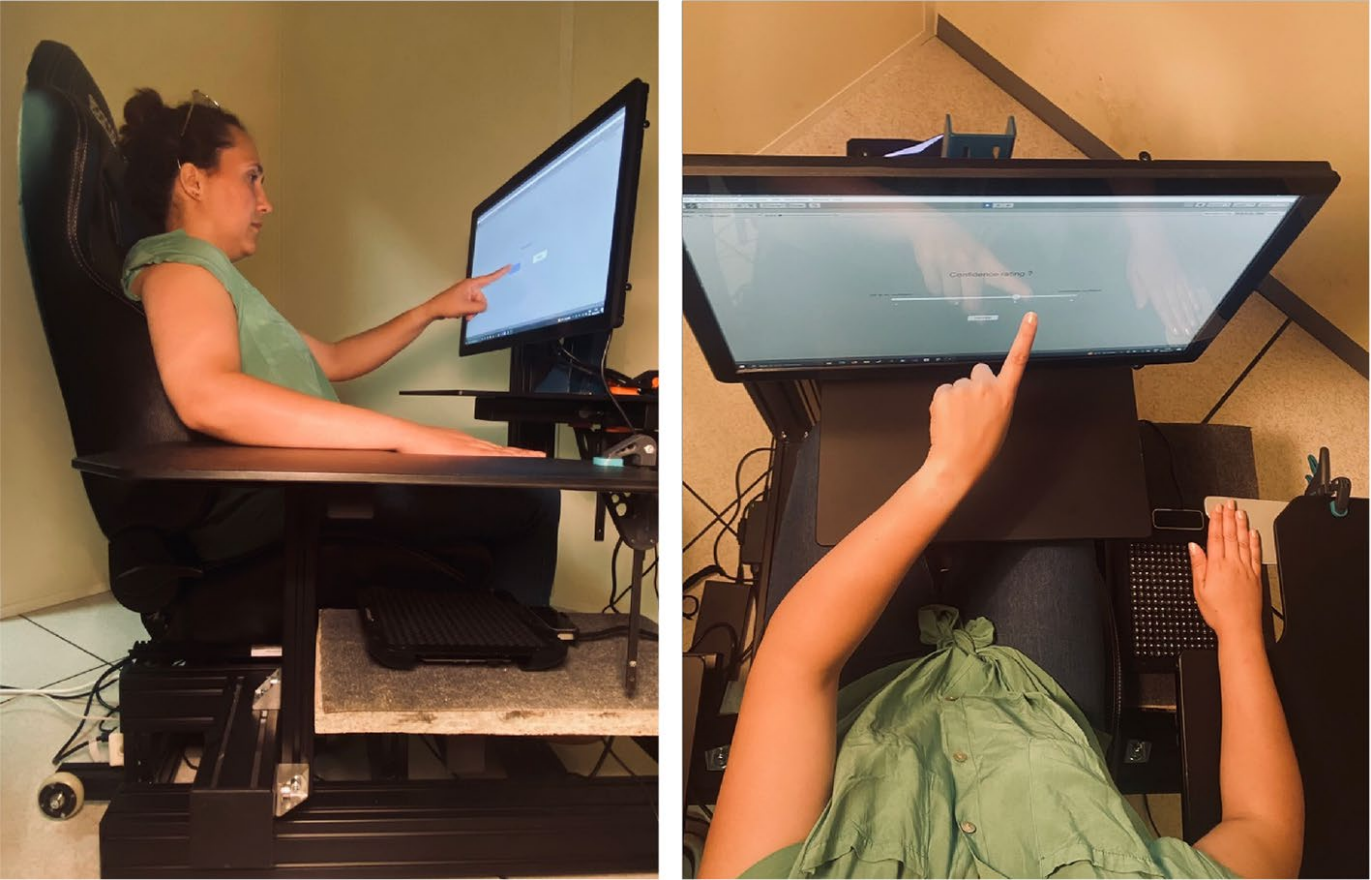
Overview of the experimental setup and participant positioning. Stimuli were delivered using an array of ultrasound actuators positioned 20 cm below the participant’s right hand.
